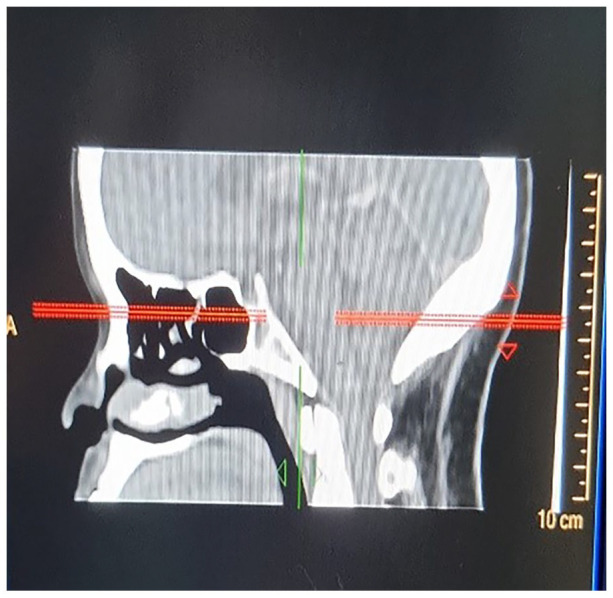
Lateral section of brain. Computed tomography (CT) image of patient’s brain.
